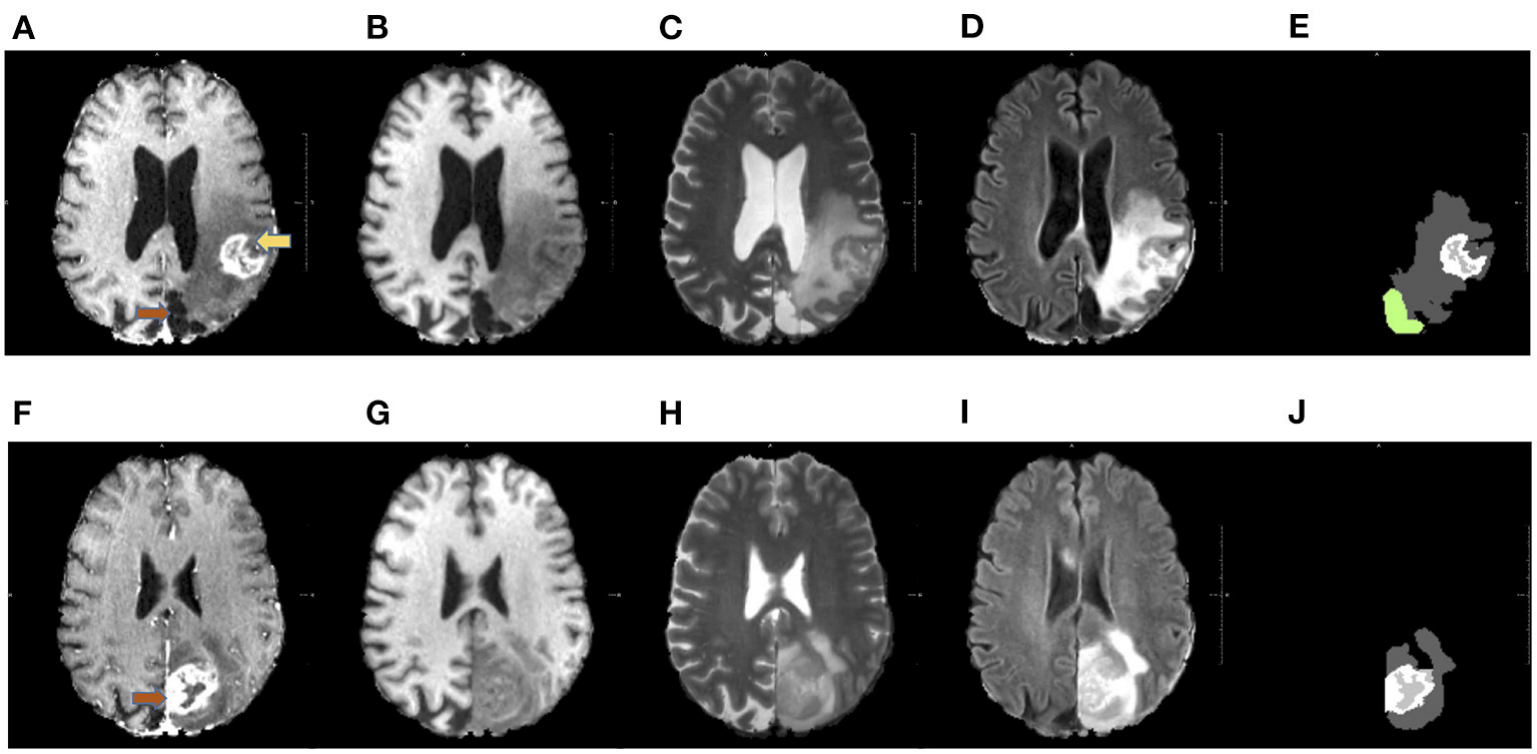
Available MRI sequences. The figures in the first row show example slices of a post-operative acquisition, whereas in the second row images of the corresponding pre-operative volume are displayed. Each volume comprehends four sequences: T1 gadolinium contrast-enhanced T1-CE in (A,F), T1 in (B,G), T2 (C,H), and FLAIR in (D,I). Each sequence is useful to spot a particular component of the pathological tissues. For example, in T1-CE the enhanced tissue is observable, whereas on FLAIR sequence the edema is well visible. The masks of the pathological tissues available for this work are visible in panels (E,J). In (E), the resection cavity is colored green. The pre-operative tumor and the corresponding resection cavity are indicated by the orange arrows. In after surgery acquisitions, pathological tissues can also be present, as in this example (the post-operative tumor is pointed by the yellow arrow).
